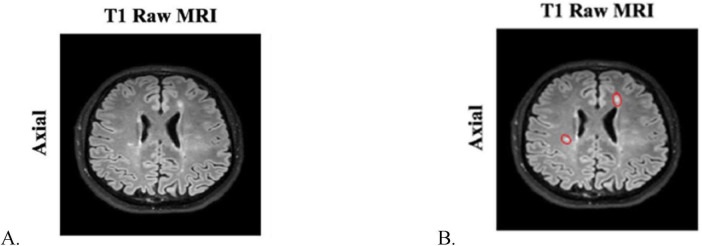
(A) Axial T1 MRI image of a person with MS lesions; (B) manually annotated MS lesions in an axial T1 MRI image (Sarica and Seker, 2022).
